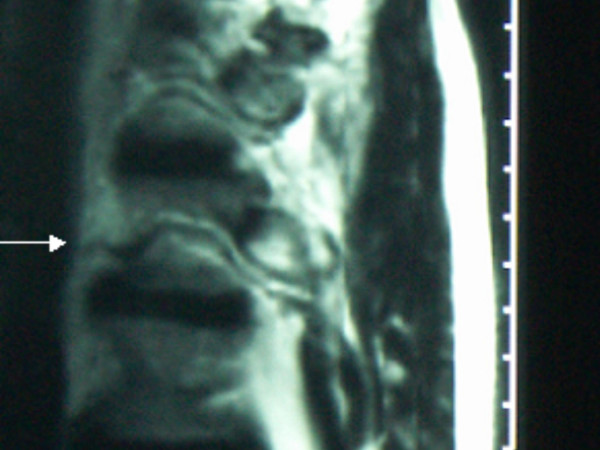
Sagittal MRI shows progressive simultaneous ossification of the anterior longitudinal and the posterior longitudinal spinal ligaments respectively and the apparent ankylosed spine fracture (arrow).
